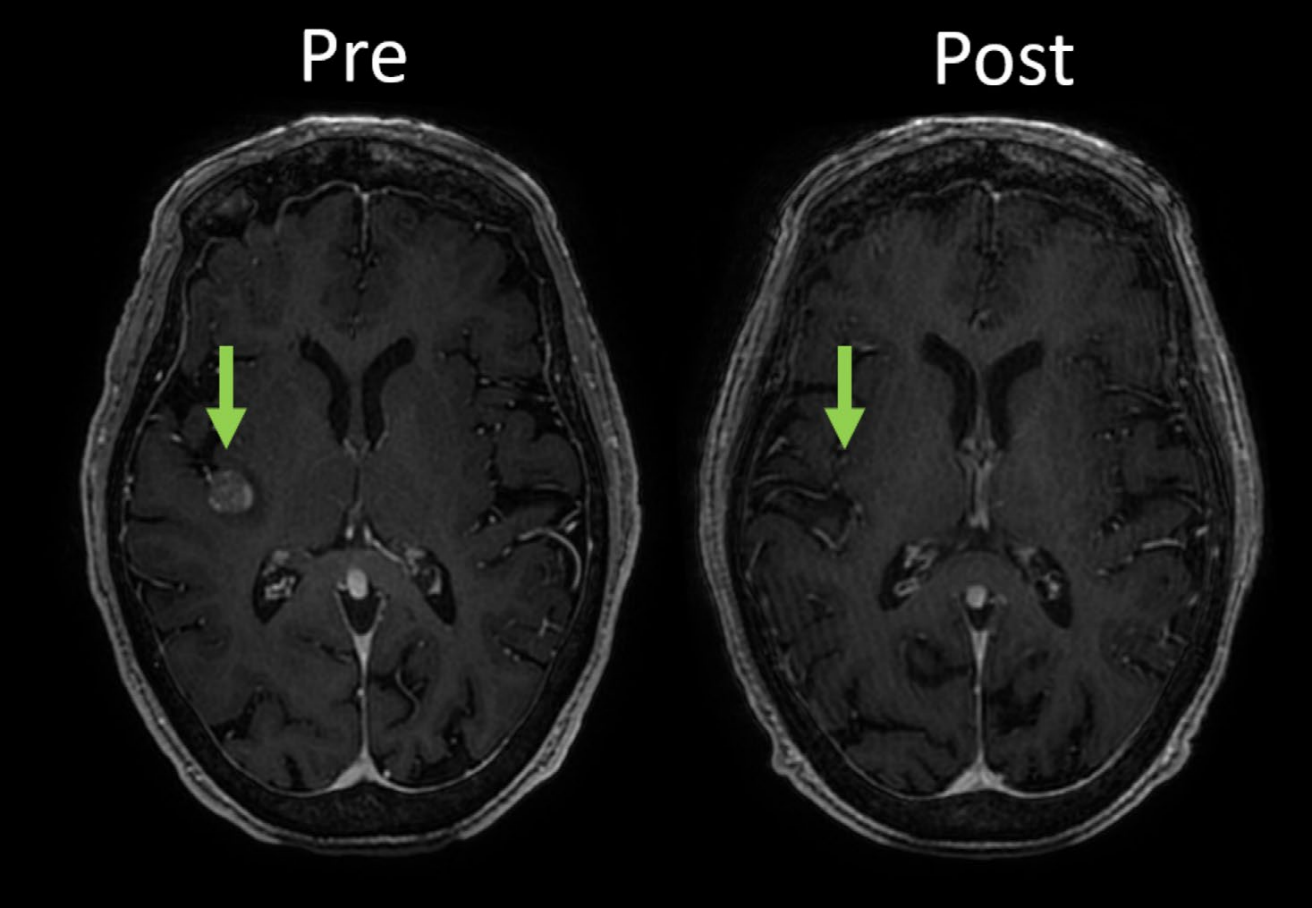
CNS efficacy in patient with SCLC. MRI of axial T1 post gadolinium images shown. Left panel, pre‐treatment. Right panel, 6 weeks post EP therapy.
